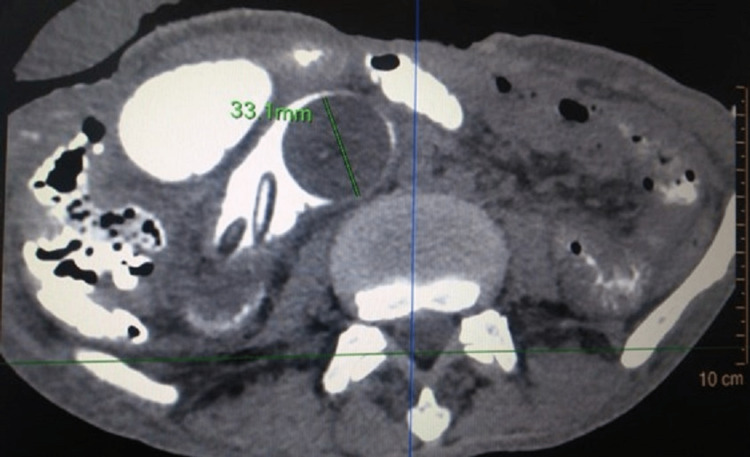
Contrast-enhanced CT showing Foley catheter bulb in the terminal ileum.
